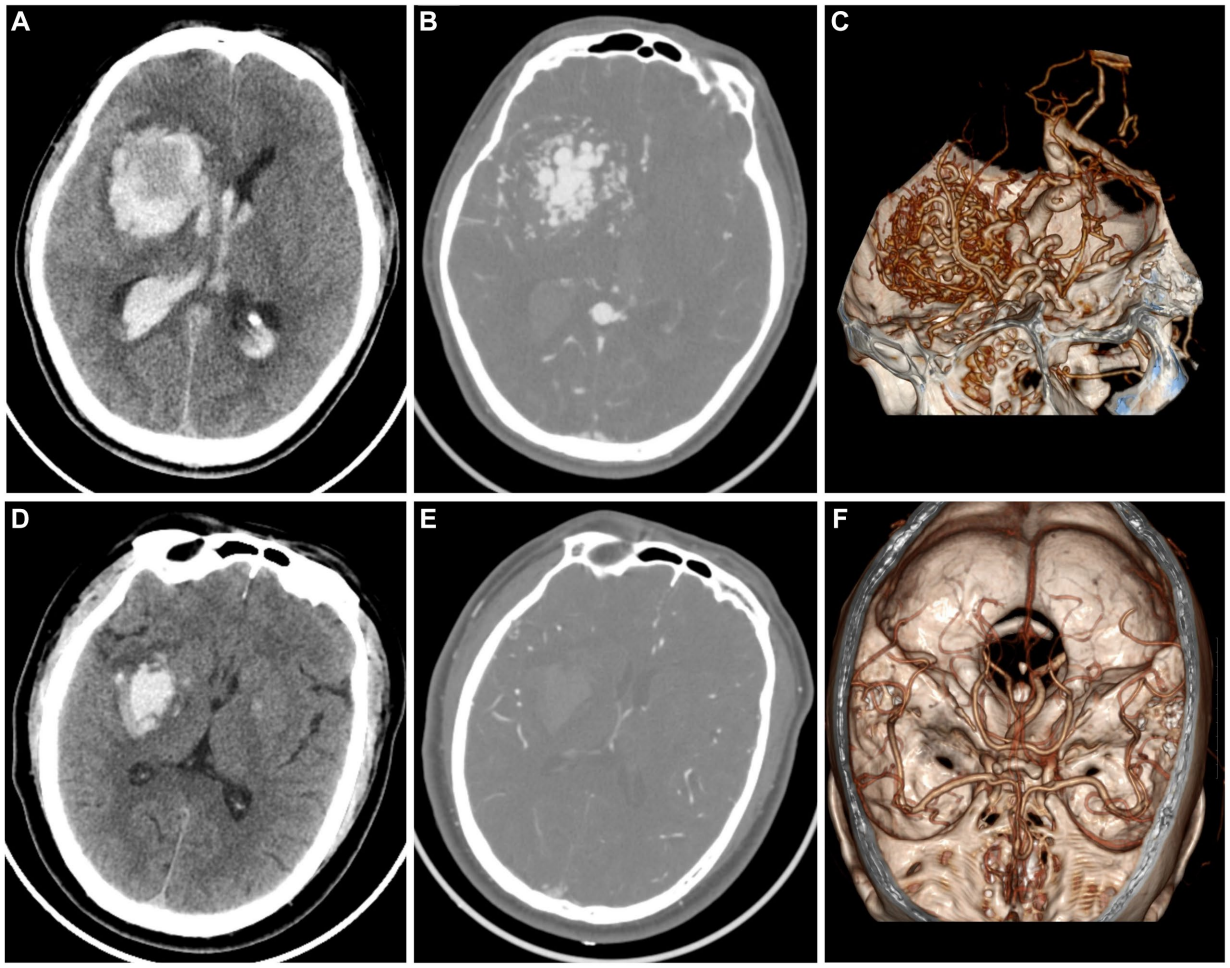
A case of AVM related hematoma, man, 42 years old, presented with unconsciousness for 4 h. Non-enhanced CT showed a hematoma in the right basal ganglia (A). CT angiography (B) and volume rendering technique (C) showed a malformed vascular mass. A case of hypertensive intracerebral hematoma, man, 47 years old, presented with slurred speech with left limb weakness for 5 h. Non-enhanced CT showed a hematoma in the right basal ganglia (D). CT angiography (E) and volume rendering technique (F) showed no abnormality.
